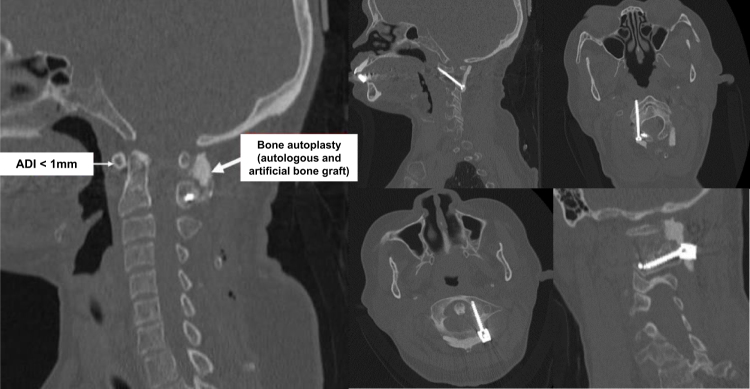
Postoperative CT scan revealed a good anatomical alignment reduction of C1–C2, with ADI reduced to 1 mm. All screws are inserted in the correct positions without penetrating the bone wall.
